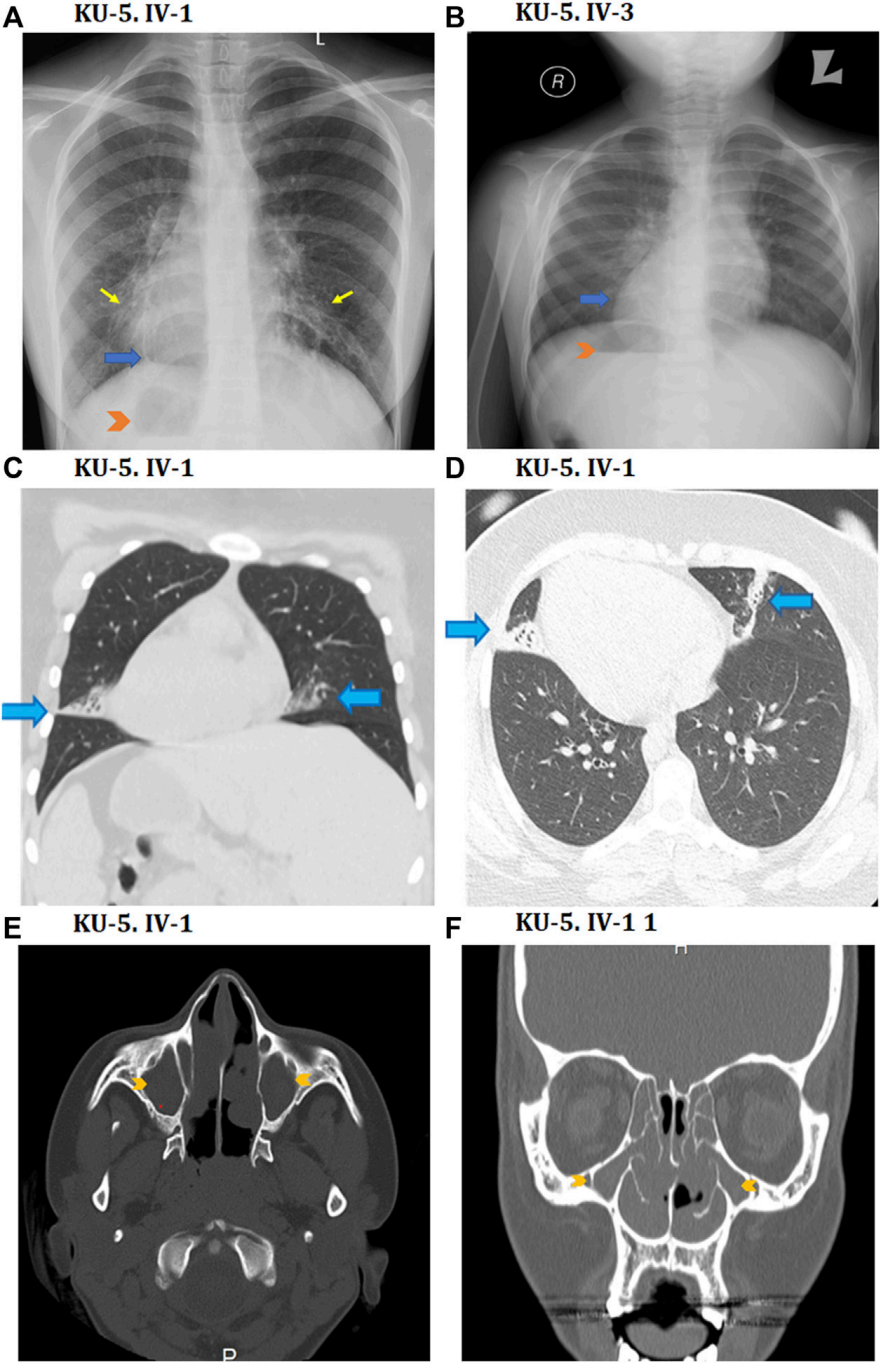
Radiological findings for the two PCD individuals of family KU-5. (A, B) Show chest X-rays for the KU-5.IV.1 and KU-5.IV.3 patients demonstrating right-sided heart apices (blue arrows) and gastric air bubbles (arrowheads) in conformity with situs inversus totalis. In addition, (A) shows mild diffuse bronchiectatic changes in the medial aspects of both lower zones (yellow arrows) for the KU-5.IV.1 patient. Selected coronal CT image in the lung window (C) as well as axial image of the lung window (D) from the CT chest study of a patient reveal situs inversus and subsegmental atelectasis in both lungs with mild underlying bronchiectatic changes (arrows). Selected axial (E) and coronal (F) reconstruction images of the CT-PNS study on the KU-5.IV.1 patient reveal complete opacification of the bilateral paranasal sinuses (arrowheads) in conformity with chronic inflammatory pansinusitis.
